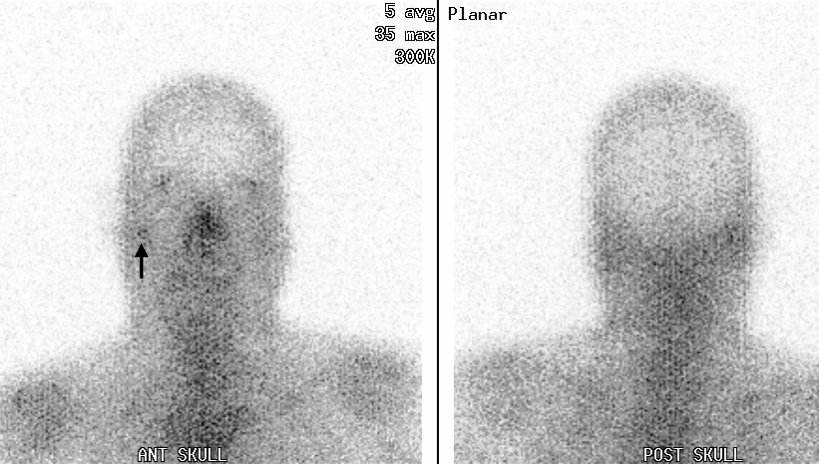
^67^Ga scintigraphy showing uptake in the right mastoid region. (black arrow).
